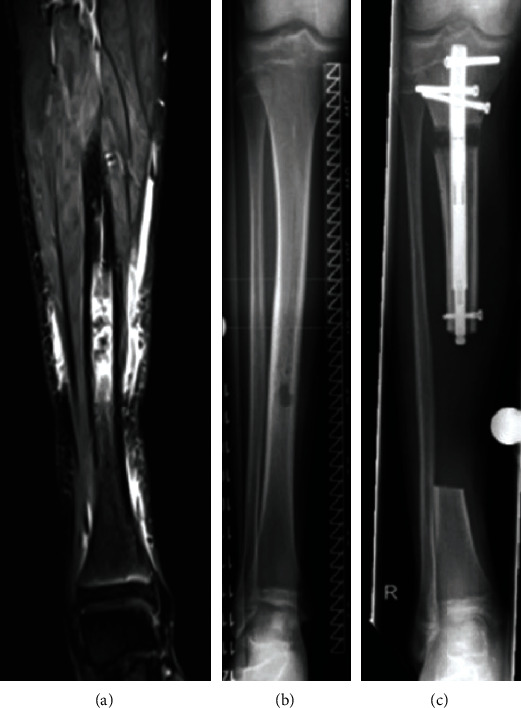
Patient 2. (a) Magnetic resonance imaging showing adamantinoma of the diaphyseal tibia. (b) Radiograph showing adamantinoma of the diaphyseal tibia. (c) Radiograph showing wide tumor resection preserving the fibula, proximal tibial corticotomy, and implantation of a lengthening nail for bone segment transport.
